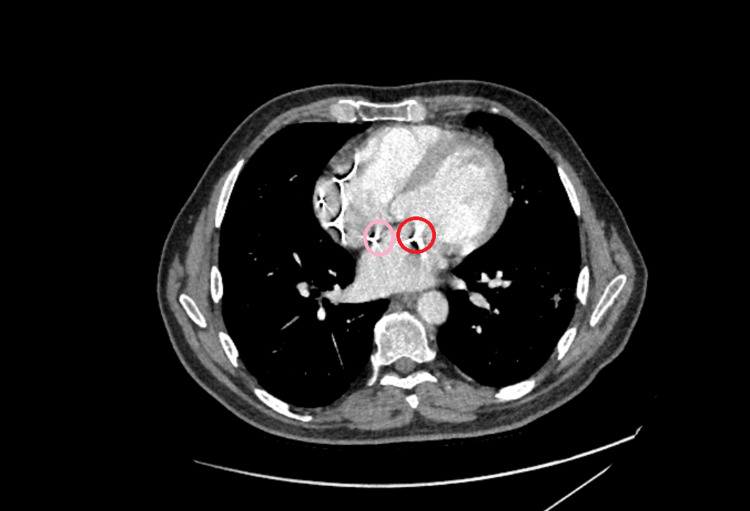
CT chest showing leads blooming artefact due to pacing lead at the level of PFO as indicated by a pink circle and mitral valve as indicated by a red circle CT: Computerised tomography; PFO: patent foramen ovale
